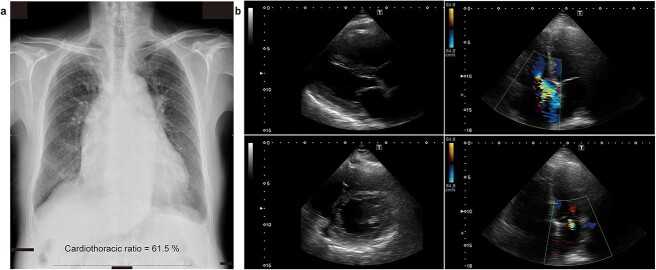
Examinations after Goreisan treatment. (a) Chest radiograph shows significant reduction in cardiac enlargement and pleural effusion. (b) Echocardiography shows decreased left atrial enlargement (upper left panel), disappearance of left ventricular D-shape (lower left panel), residual severe tricuspid regurgitation (upper right panel), and reduction in mitral regurgitation (lower right panel).
